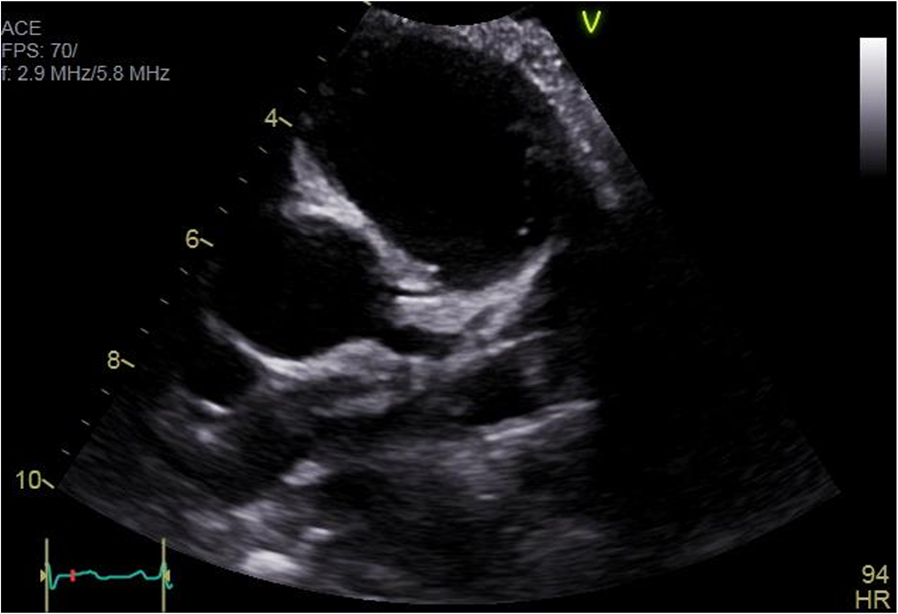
Echocardiogram. Initial echocardiogram demonstrating dilation of the left main coronary artery (5.32 mm, Z score + 4.25)
